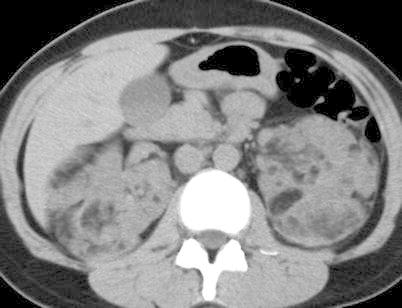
Angiomyolipoma in a 32-year-old woman with tuberous sclerosis complex. Transverse, unenhanced CT (5-mm sections) shows multiple bilateral renal masses each containing fat attenuation (less than −10 HU) diagnostic of angiomyolipomas.
